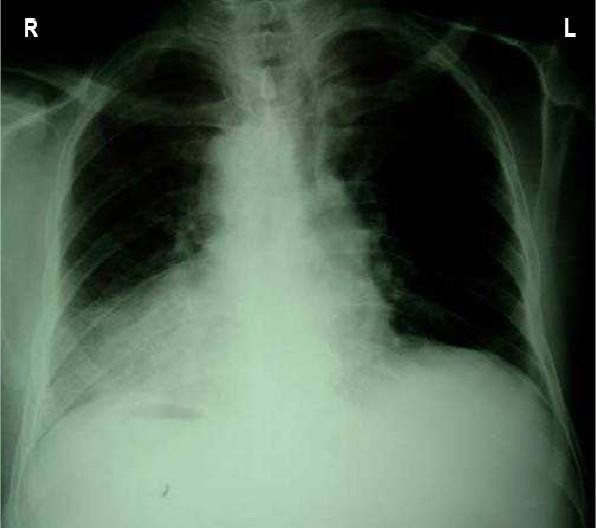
Chest X-ray demonstrating dextracardia.
